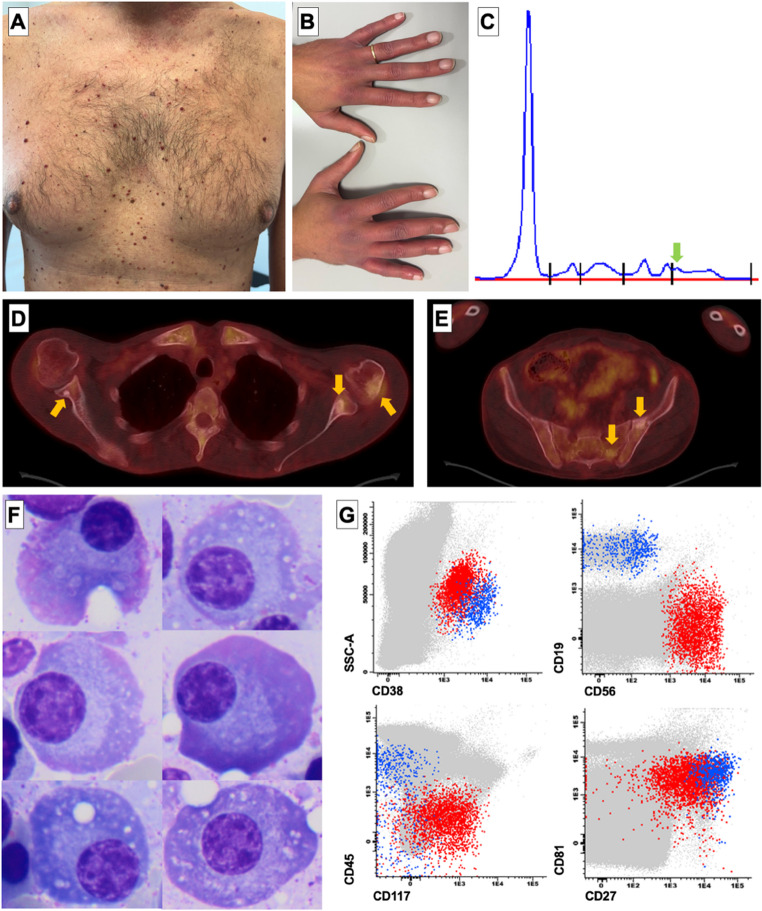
Patient findings before treatment with ARI0002h. (A): Cutaneous angiomas. (B): Raynaud syndrome. (C): Monoclonal IgA-lambda migrating in the gamma region on serum electrophoresis (arrow). (D-E): PET-CT showing sclerotic lesions in multiple bones, including humeri, scapulae, iliac pale, and sacrum (arrow), some of them with FDG-18 uptake. (F): Bone marrow aspirate assessment at 100x magnification, showing multiple aberrant plasma cells, some of them with flame cell morphology. (G): Plasma cells immunophenotype comparing normal plasma cells (blue) and aberrant plasma cells (red). Aberrant plasma cells showed CD38^dim^, CD45-, CD19-, CD56+, CD117^dim^, CD81+
